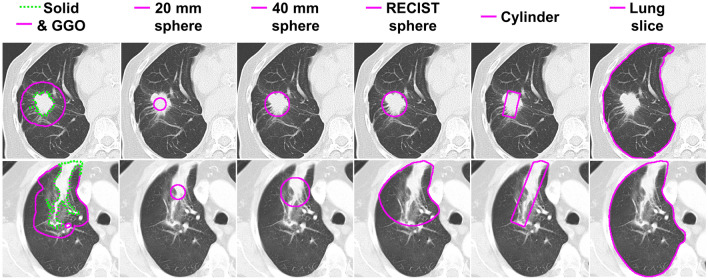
An axial slice through the six regions of interest we tested in our study for two tumors, one on each row, shown on the CT scan [window: 1500 HU, level: − 600 HU] with RECIST recurrence.
